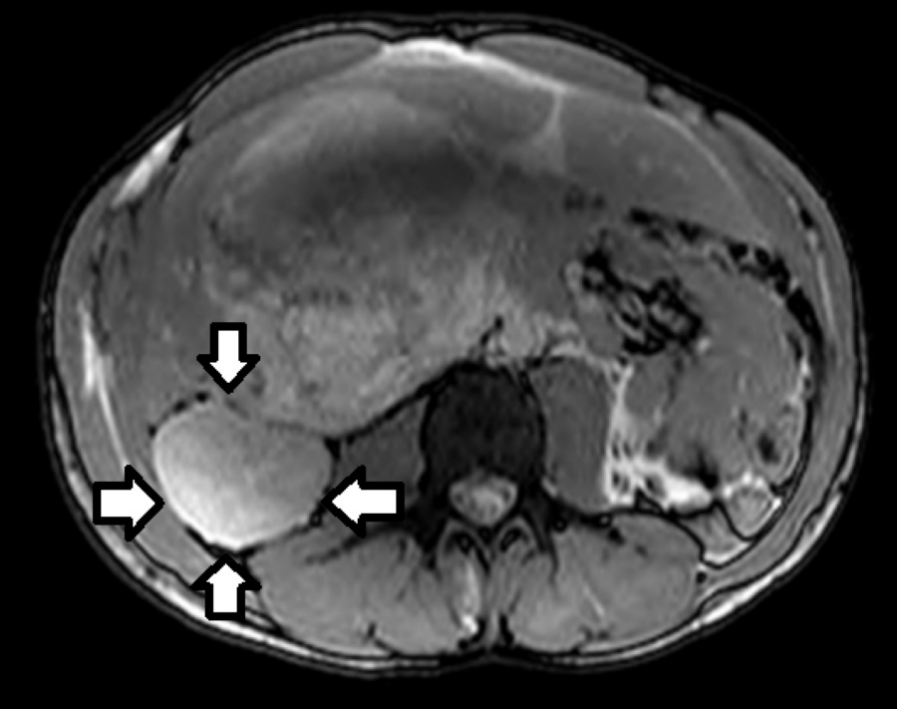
MRI showing caudal lesion of undifferentiated embryonal sarcoma of the liver MRI: Magnetic resonance imaging The caudal lesion of undifferentiated embryonal sarcoma of the liver has been highlighted by the arrows.
